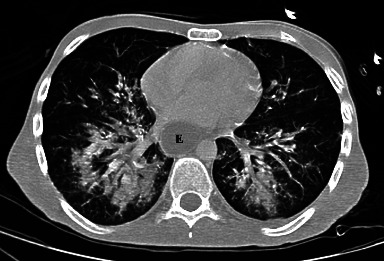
Axial CT scan of the chest with maximal intensity projection (MIP) reconstruction showing bilateral multifocal parenchymal condensations with multiple ground-glass opacities predominant in the lower lobes, suggestive of aspiration pneumonia. It also shows important distension of the esophagus (E).
